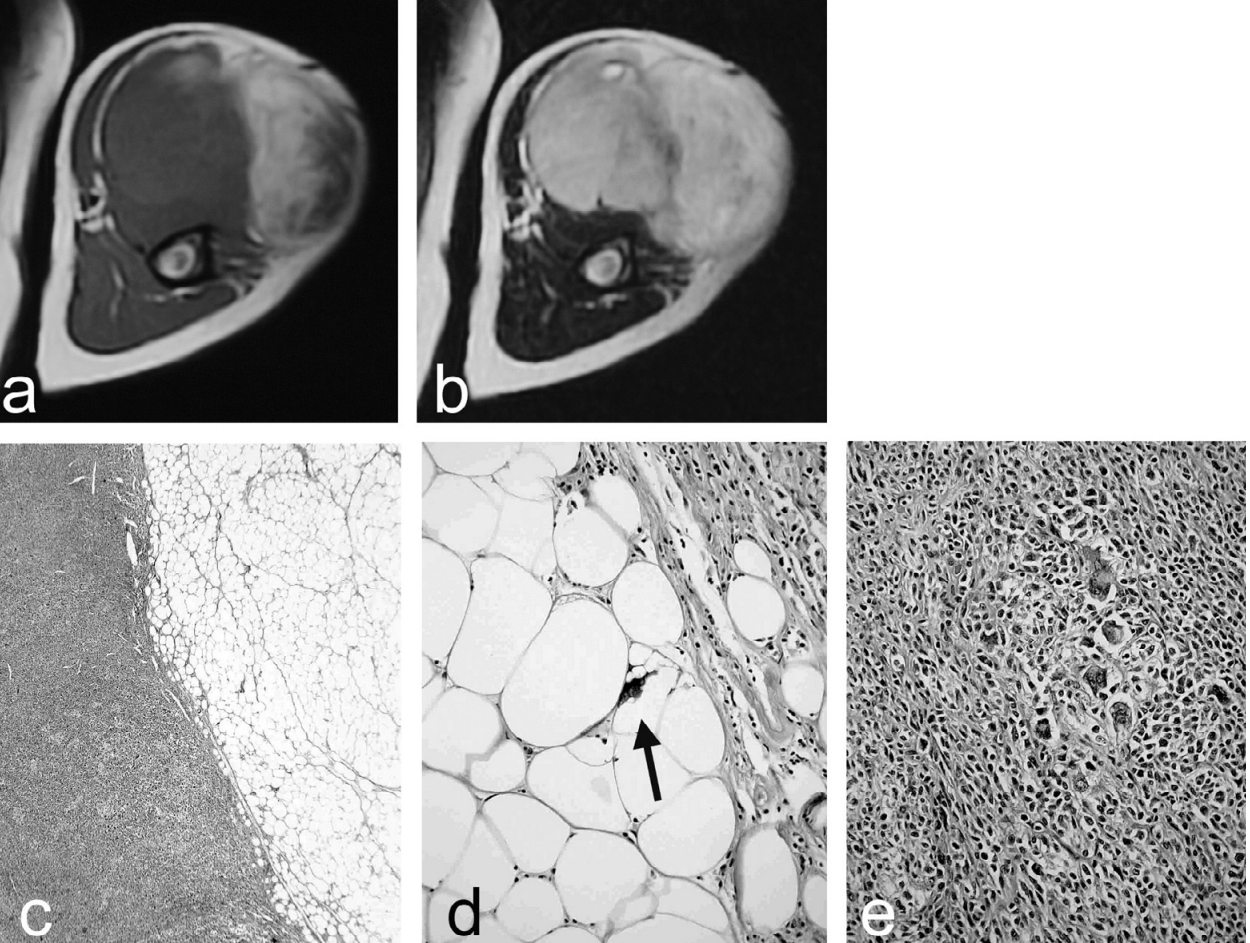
MRI shows a tumor with two components of high T1-weighted (a) and high T2-weighted images (b) (right portion; well-differentiated components), and of low T1-weighted (a) and high T2-weighted images (b) (left portion; dedifferentiated components). Well-differentiated components and dedifferentiated components with a distinct border (c). Well-differentiated components show well-differentiated lipoma-like liposarcoma with lipoblasts (arrow) (d). Dedifferentiated components are composed of atypical spindle cells in short fascicles, resembling MFH (bottom; left) (e).
